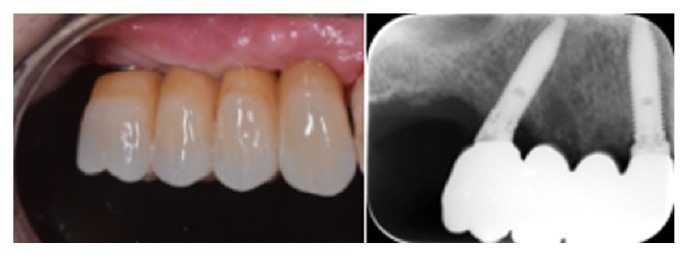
Vestibular and radiographic views of definitive restoration at 36-month follow-up.
